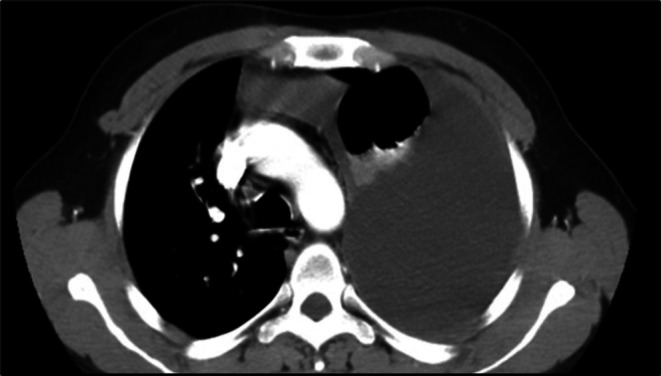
Chest CT scan 1 month after starting TB treatment 26 August 2022 demonstrating the left side massive pleural effusion.
